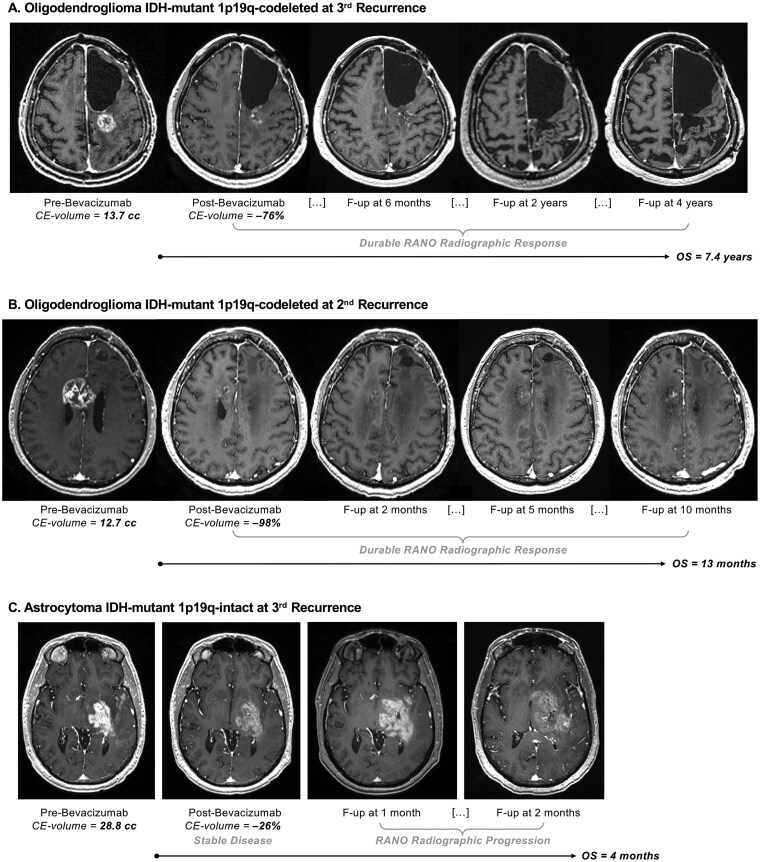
Representative cases of post-bevacizumab radiographic response patterns. Patients (A and B) with a smaller pre-bevacizumab CE tumor volume, deeper post-bevacizumab tumor shrinkage, and RANO durable radiographic response tend to have longer OS compared to patients (C) without these favorable imaging findings. For each patient, images were selected to display the slice with the largest tumor area at each time point.
